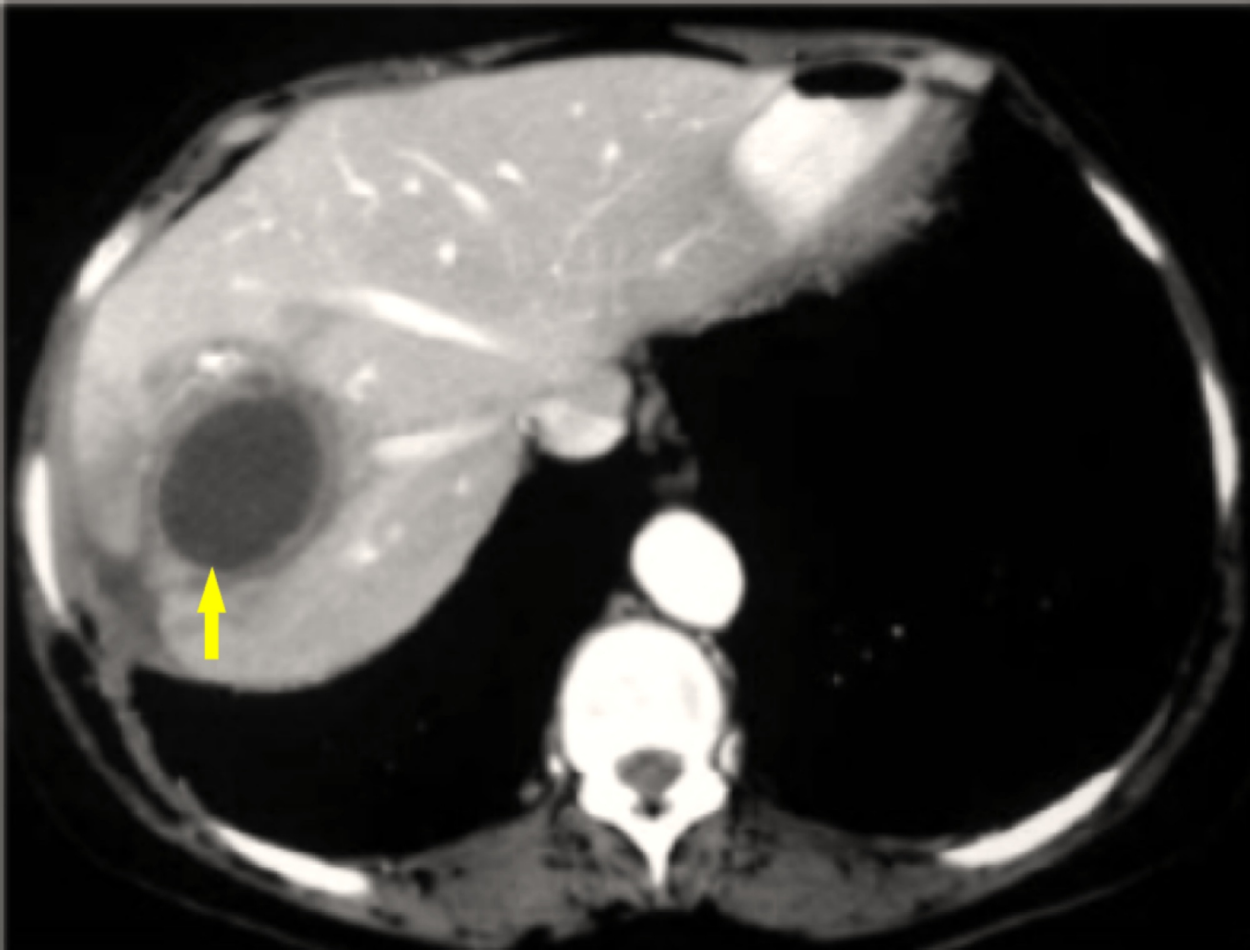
CT scan with contrast shows fluid collection in the right lobe of the liver surrounded by the rim of hypoattenuation (yellow arrow).
